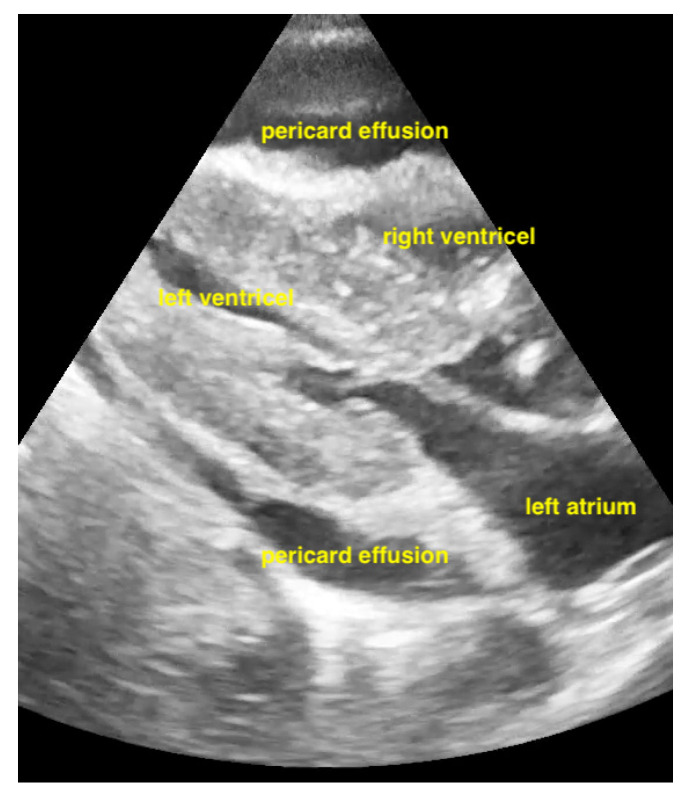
Pericardial effusion in parasternal long-axis view.
